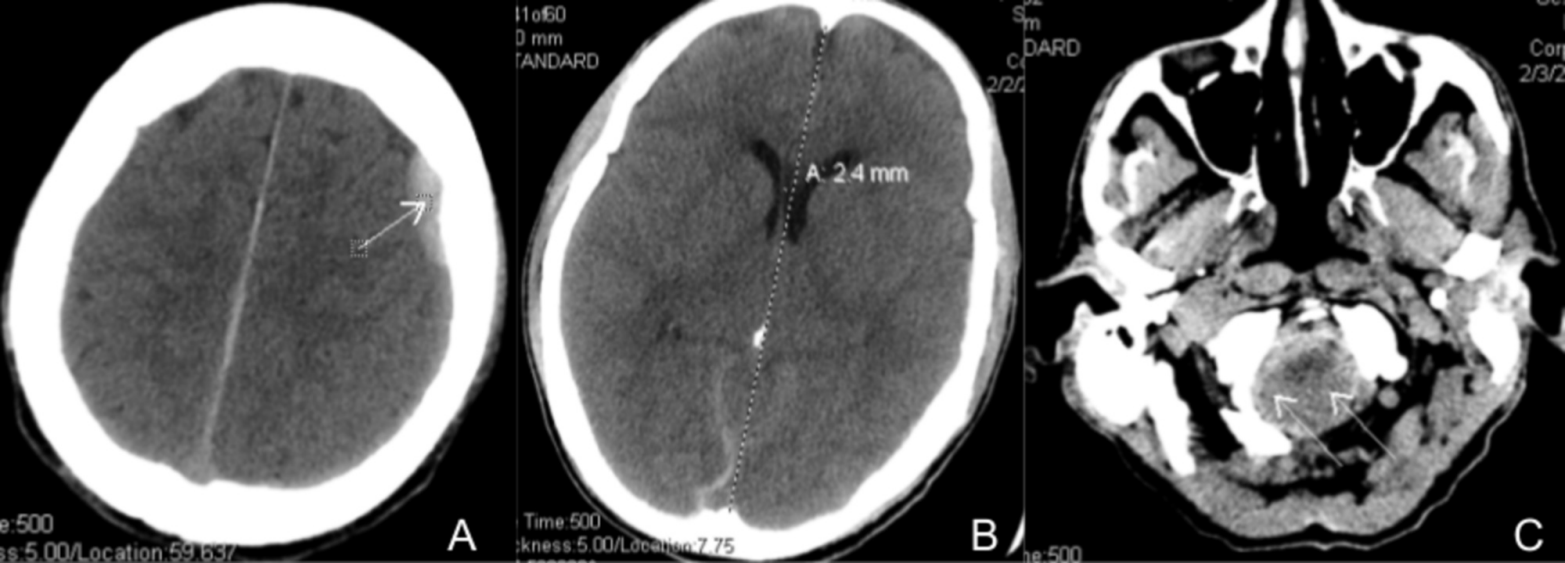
Brain computed tomography (CT) without contrast A) Worsening subdural hemorrhage (arrow); B) worsening mass effect with midline shift; C) low-lying cerebellar tonsils crowding the foramen magnum (double arrow)
